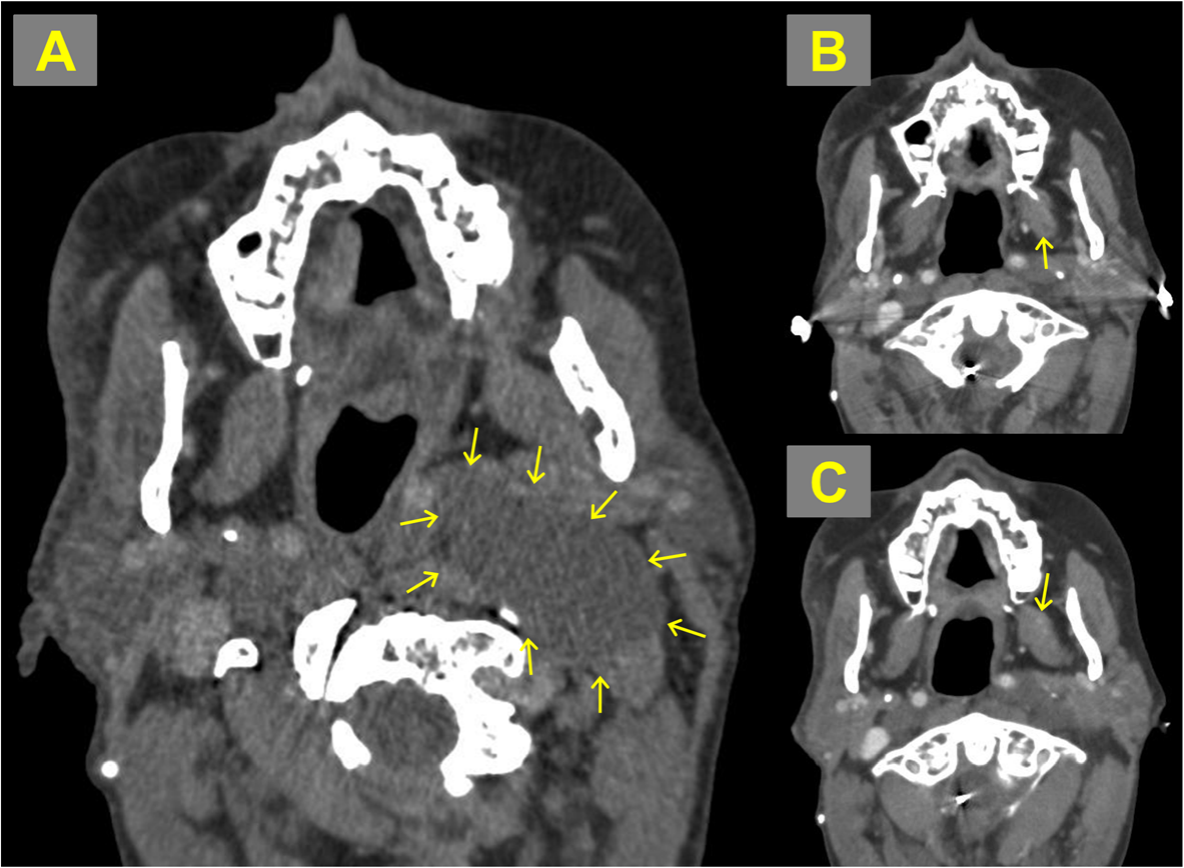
Axial MRI scanning of neurofibroma of the neck. A: Baseline MRI showing a large lesion of the left neck compressing the nearby hollow organs, causing a slight breathing and swallowing compromise. B and C: three months post-PDT scans, slightly upper and lower to the pre-PDT scan level, showing significant shrinkage with an increase in airway patency.
